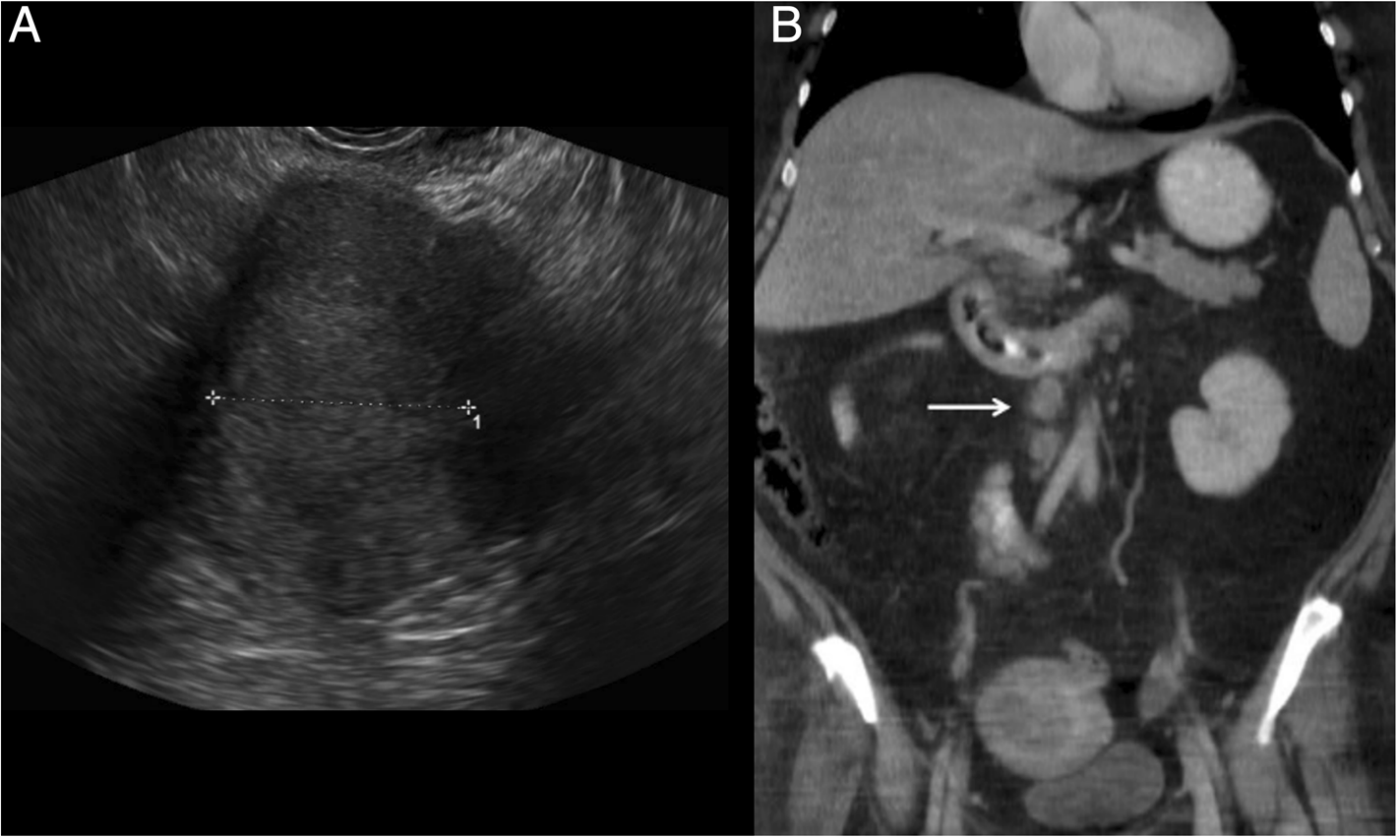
A 46-year-old woman with EC grade 3. TVUS image (a) shows an endometrial mass of 4.79 cm (measure in yellow) invading < 50% of the myometrium. Despite a superficial myometrial invasion, CT images at staging (b) showed pelvic and lombo-aortic lymphoadenopathies (arrow), that bwere confirmed at surgery
